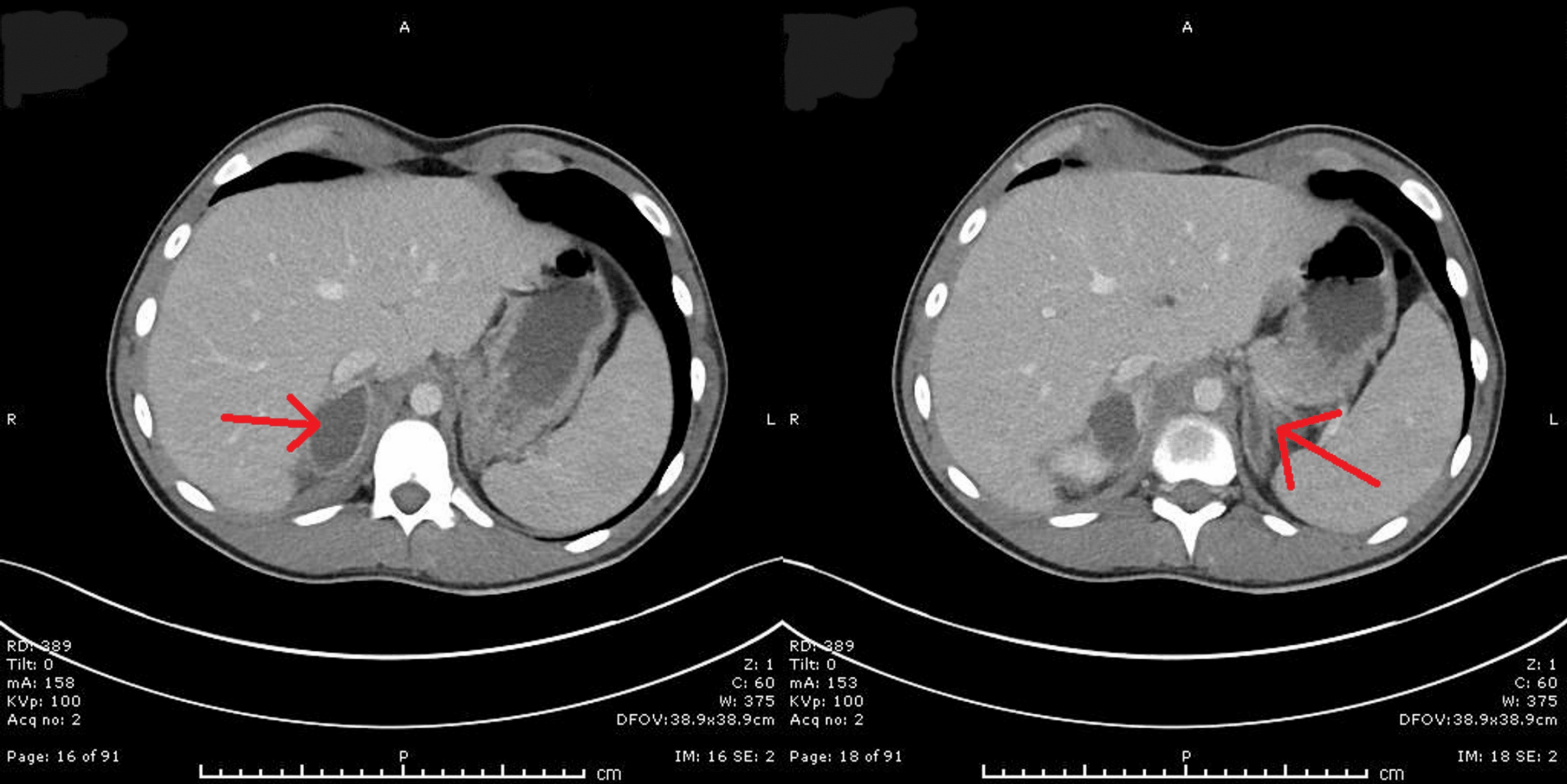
Contrast-enhanced CT abdomen demonstrating right adrenal abscess (left red arrow) and left adrenal abscess (right red arrow)
